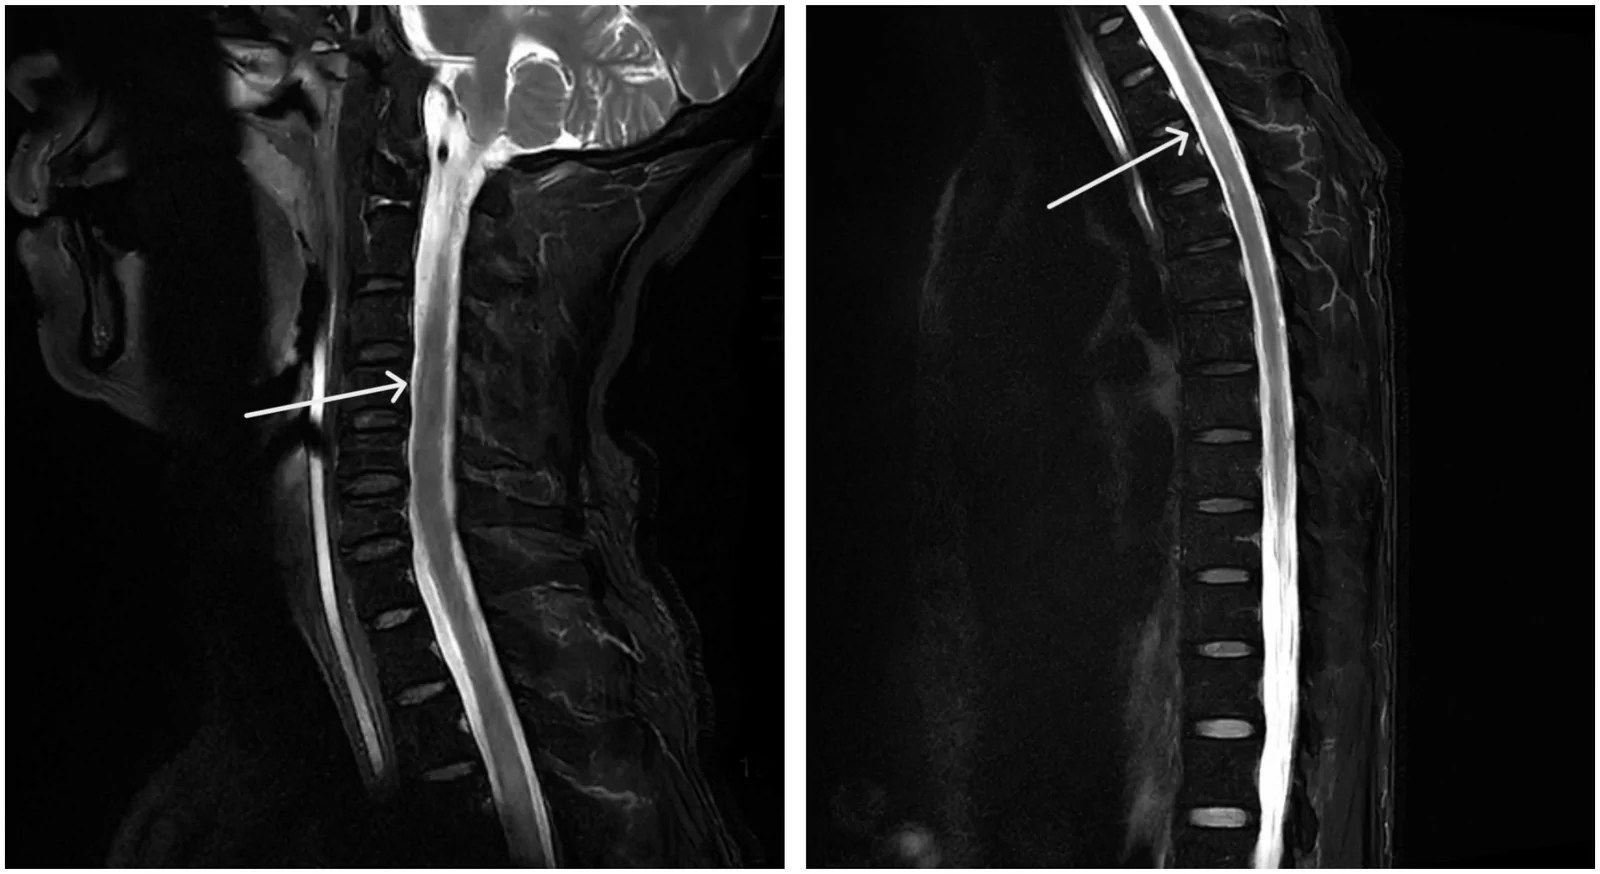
Magnetic resonance imaging of the cervical and thoracic spine of patient 3. T2-weighted imaging shows hyperintensity in the C2, C4, C5, and T2-T4 vertebral body levels, suggesting inflammatory lesions.
